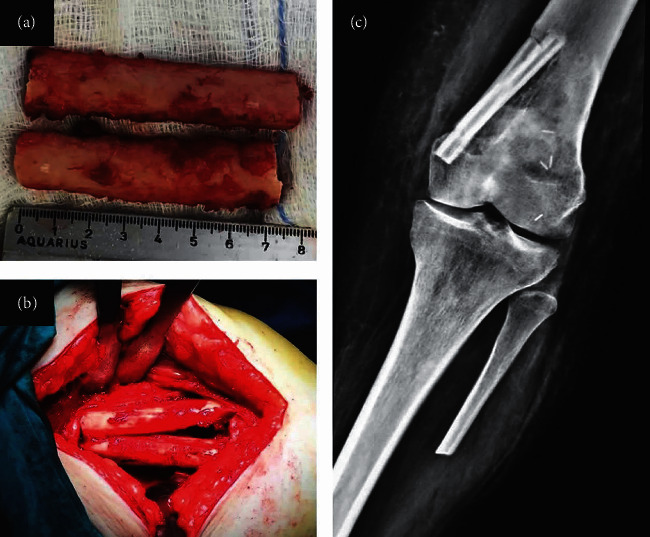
(a) Autograft from the contralateral fibula was used and was cut in the longitudinal axis. (b) Two pieces of hemicortical fibula were inserted to the posterior-medial part of the distal femur after excision of the PMMA. (c) Postoperative anteroposterior radiographs of the knee showing the fibula autografts in place.
